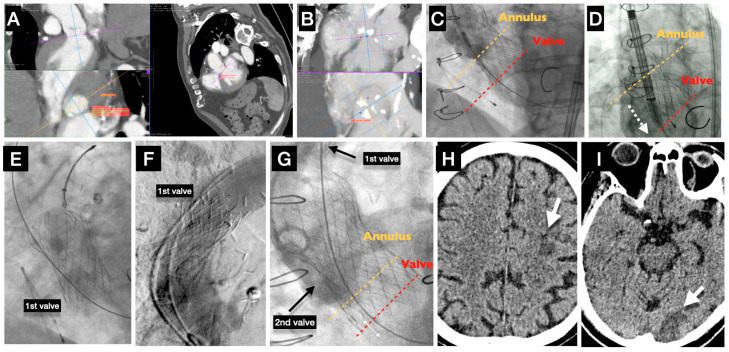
A case example of an acute stroke in a patient who had a rare TAVI complication. (A): preprocedural CT evaluation showed a calcified degenerative aortic valve stenosis, suitable for a Medtronic EvolutR, 29 mm. (B): distribution of calcium in native valve’s cusps. (C): The first valve deployment failed because it migrated to the LV with severe aortic valve regurgitation. (D): Attempt to insert a second valve complicated by pushing the first valve almost completely into the LV (dotted arrow). (E): The first valve was successfully snared up to the ascending aorta. (F): The first valve was retrieved and left at the level of the brachio-cephalic trunk. (G): The second valve was successfully implanted at the right position without regurgitation. (H,I): The next day, the patient complained of blurred vision. Two recent ischemic stroke lesions were identified in brain CT (white arrows).
